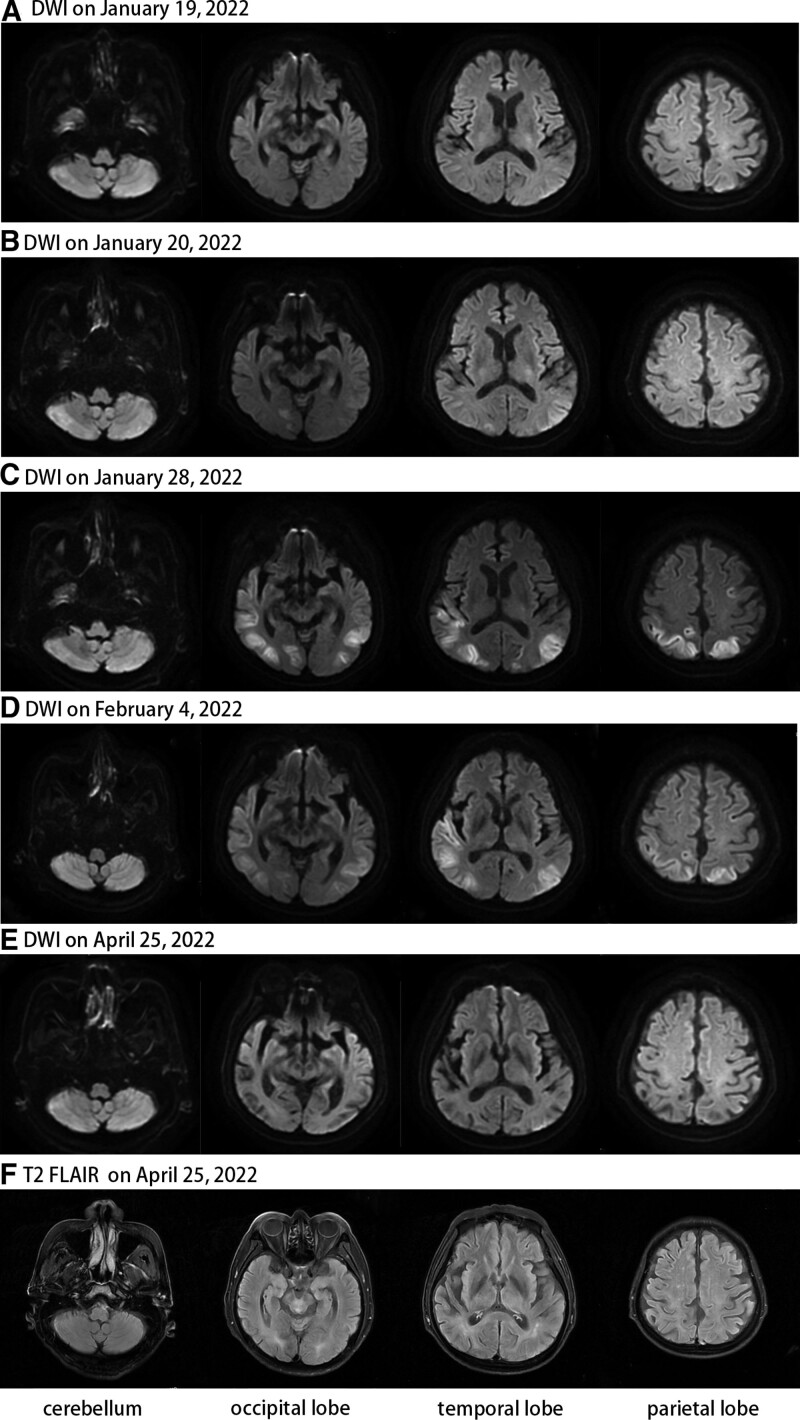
Magnetic resonance images during the first hospitalization. (A) DWI on January 19. No obvious hyperintensities were seen. (B) DWI on January 20. The bilateral cerebellar and occipital lobe have patchy slight hyperintensities. (C) DWI on January 28. The bilateral parietal lobe, occipital lobe, and temporal lobe had multiple patchy hyperintensities, which were larger than the last time, and some of them appeared new. (D) DWI on February 4. Hyperintensities are lower than last time. (E) DWI on April 25. No obvious hyperintensities. (F) T2 FLAIR on April 25. No obvious lesions left. DWI = diffusion-weighted image, FLAIR = fluid-attenuated inversion recovery.
